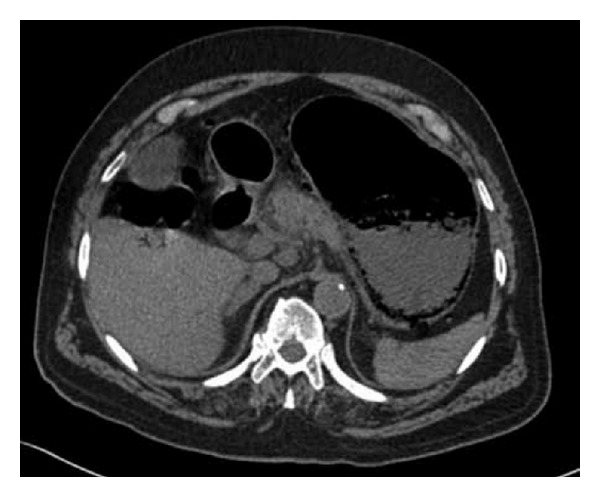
CT showing gas within the posterior gastric wall, indicative of gastric ischaemia (Case 1).
